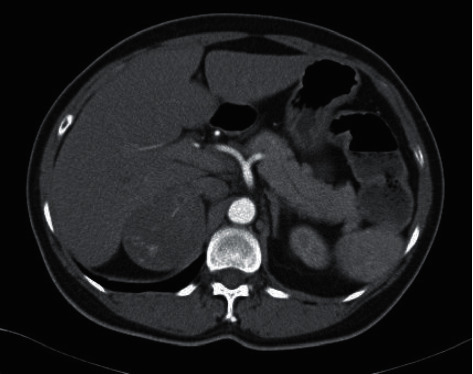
CT abdomen of Patient A on 9 August 2012. A 6.4 × 5.2 × 5.8 cm heterogeneously enhancing mass in the right adrenal gland with pre-contrast HU 35.4 (red arrow).
